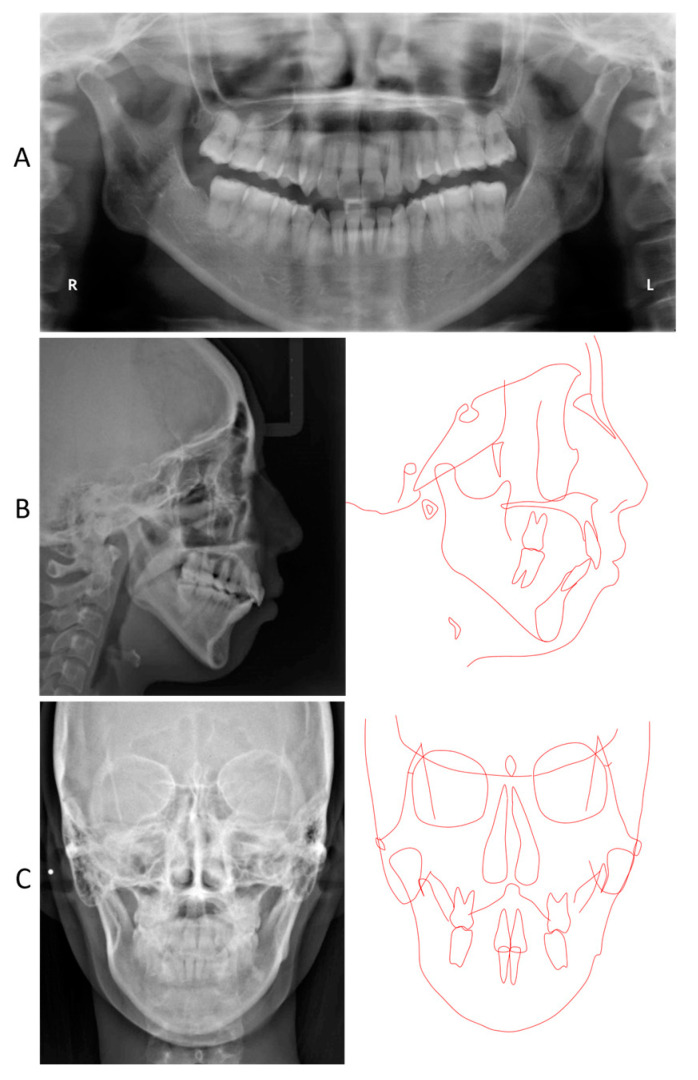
Posttreatment radiographical records. (A) Panoramic radiograph; (B) Lateral cephalogram and its tracing; (C) Frontal cephalogram and its tracing.
